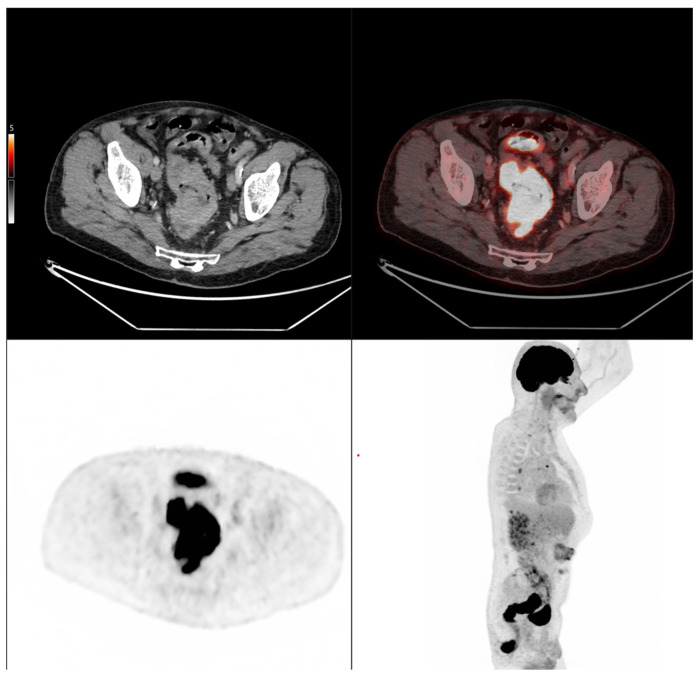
Case 2: A 69-year-old patient with stage IIA rectal cancer, presenting with a semi-circumferential primary tumor with craniocaudal extension and MTV both below the predictive cut-offs (5.1 cm vs. 6.2 cm; MTV 10.1 vs. 35.49 for OS and 32.08 for PFS). Baseline FDG PET/CT (top row) revealed intense hypermetabolism (SUVmax 38.8). Interim FDG PET/CT (bottom row) showed a good partial metabolic response, and the patient experienced sustained clinical benefit with no progression in the long term (OS = PFS = 29 months).
